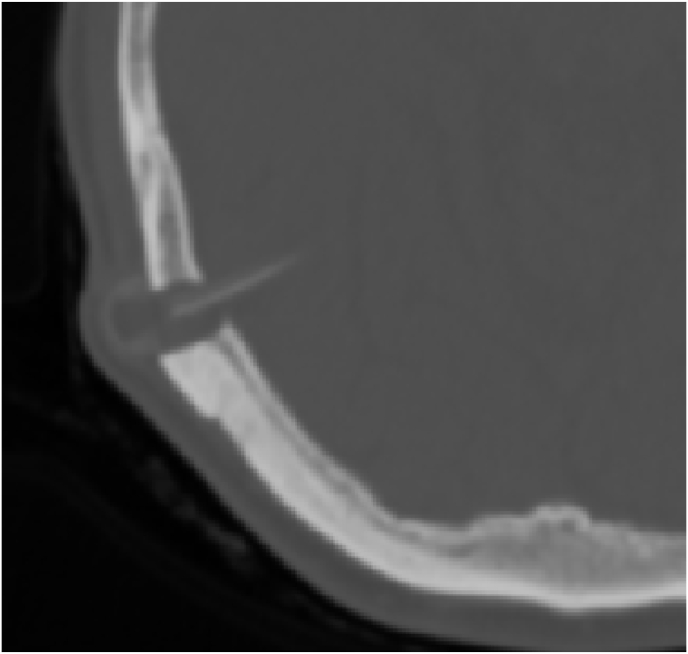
Control CT scan 1 month later revealed the regression of the bubble air.
